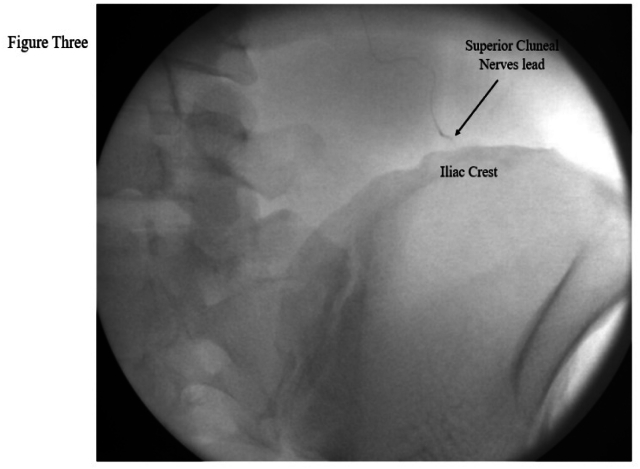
Xray AP View *Shows the lead location.*
